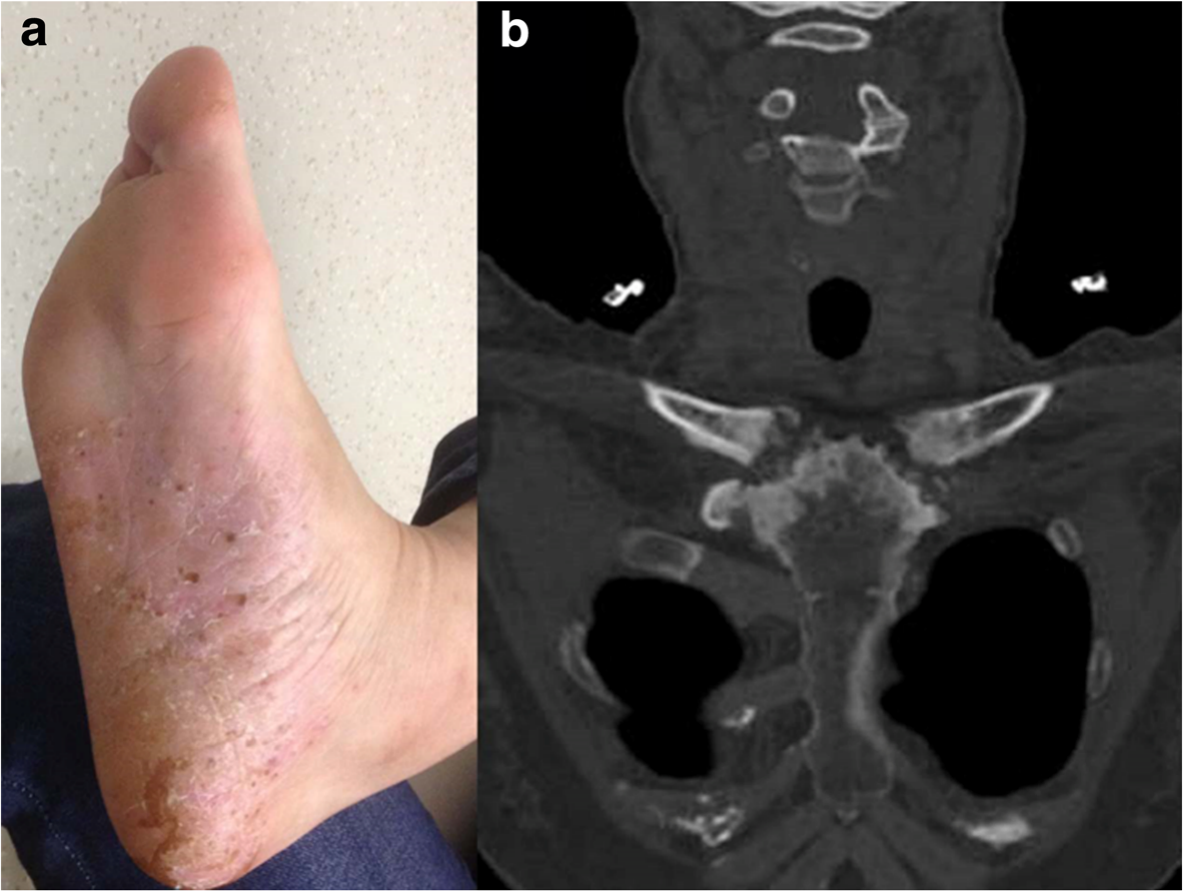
A 50-year-old woman with SAPHO syndrome presented with recurrent palmoplantar pustulosis for seven years and multiple joint pain for six years. (a) Erythema with pustules and scales on the right foot. (b) Coronal CT image of sternoclavicular joints demonstrates hyperostosis and erosive changes
